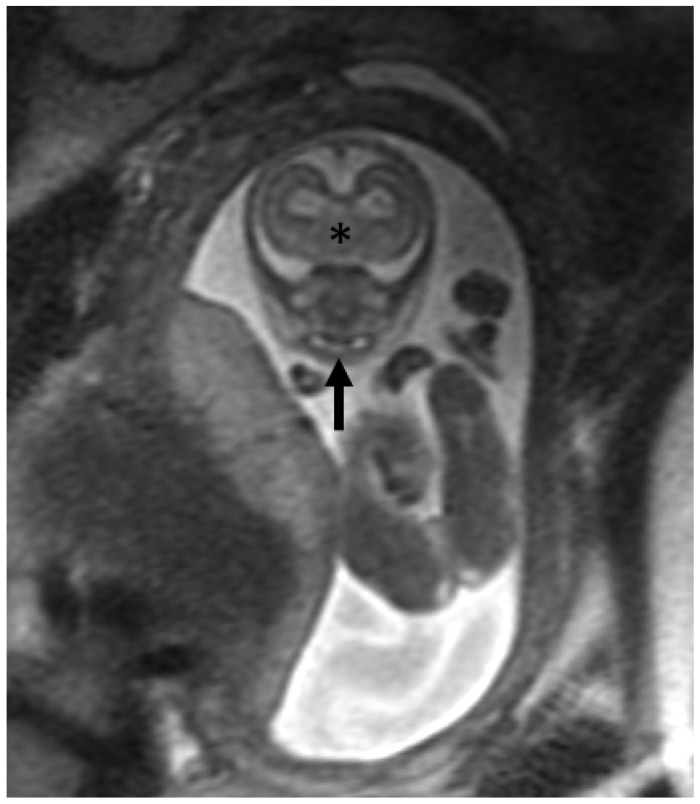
Fetal T2-weighted MRI showing single central incisor of a patient (up arrow) and the failed thalamic separation (*) compatible with holoprosenchephaly.
